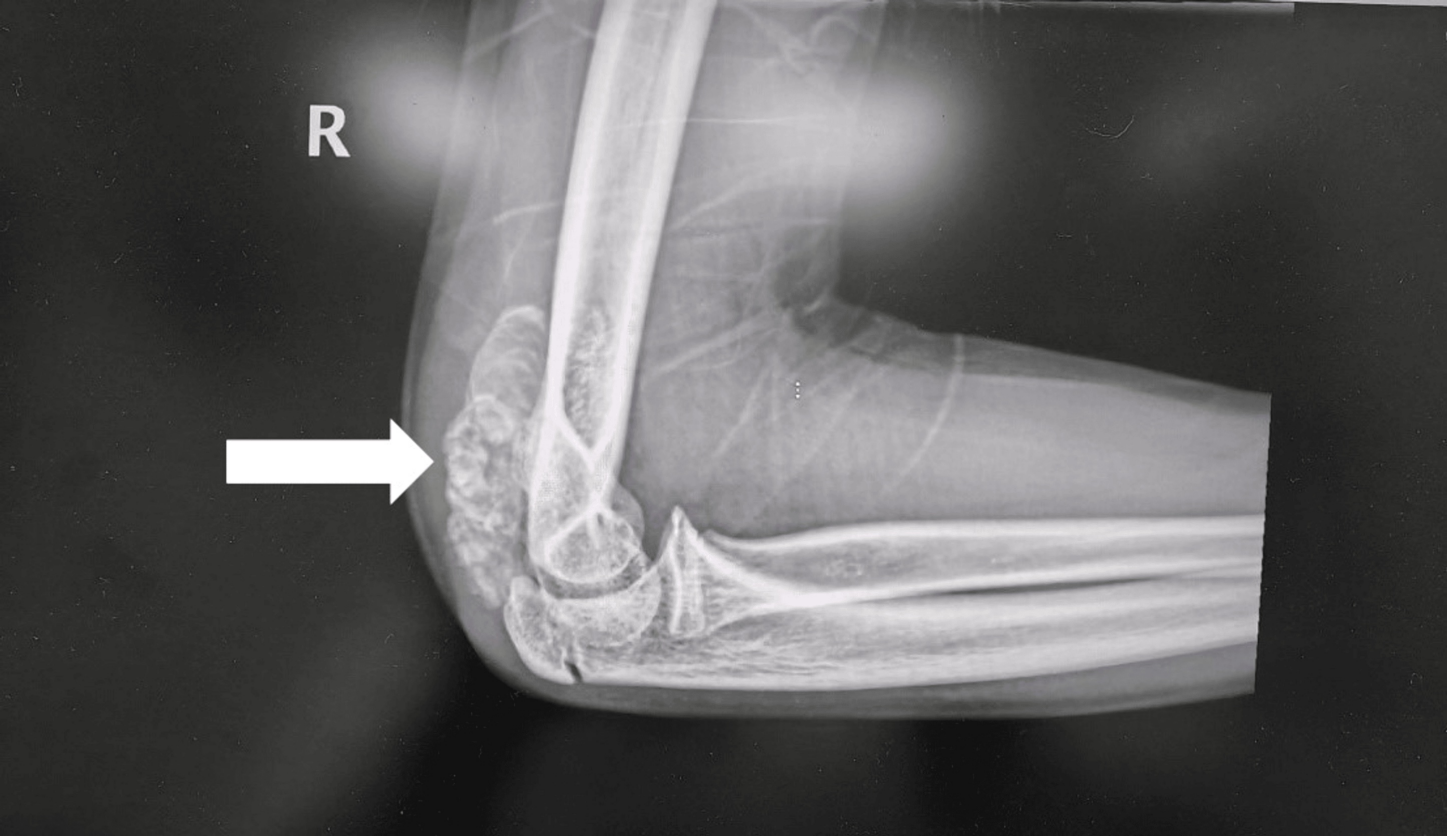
Lateral X-ray of right elbow shows right elbow periarticular calcification (arrow).
